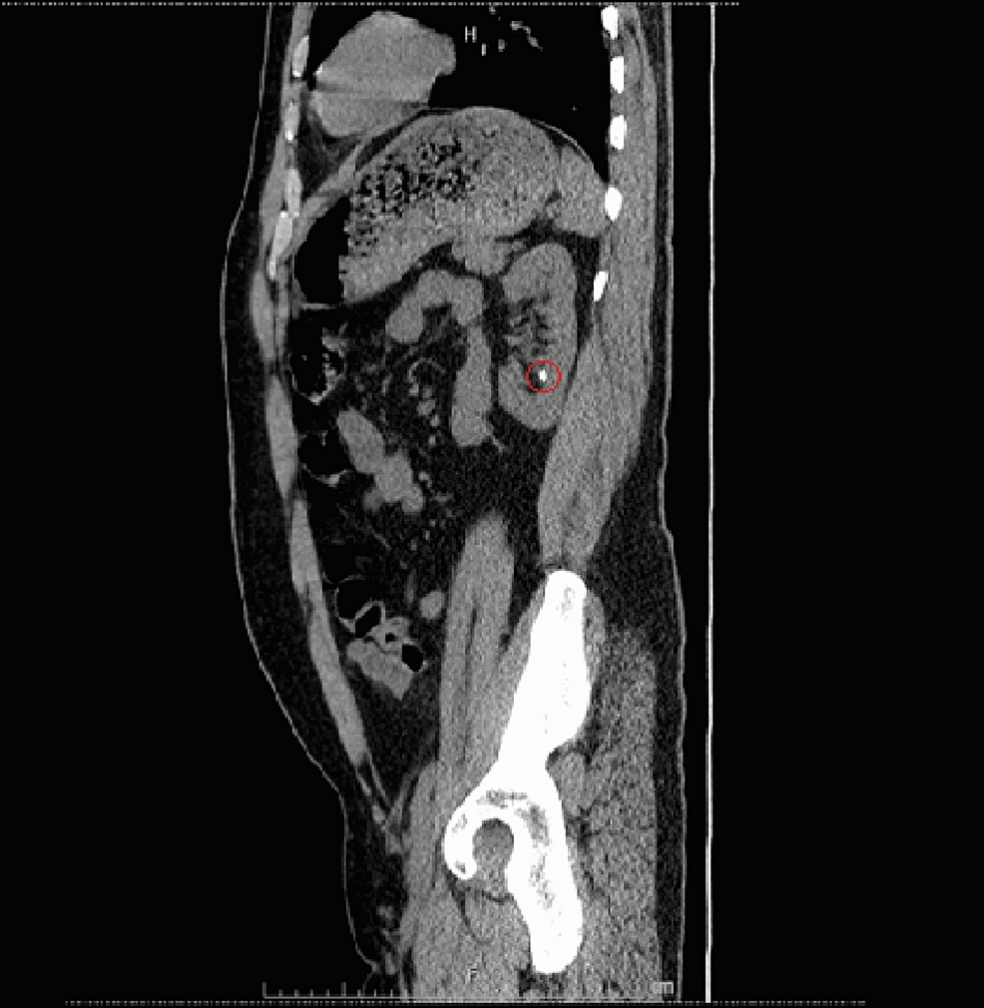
Sagittal view of CT abdomen pelvis without IV contrast. Red circle marking 6 mm non-obstructing left renal stone.
